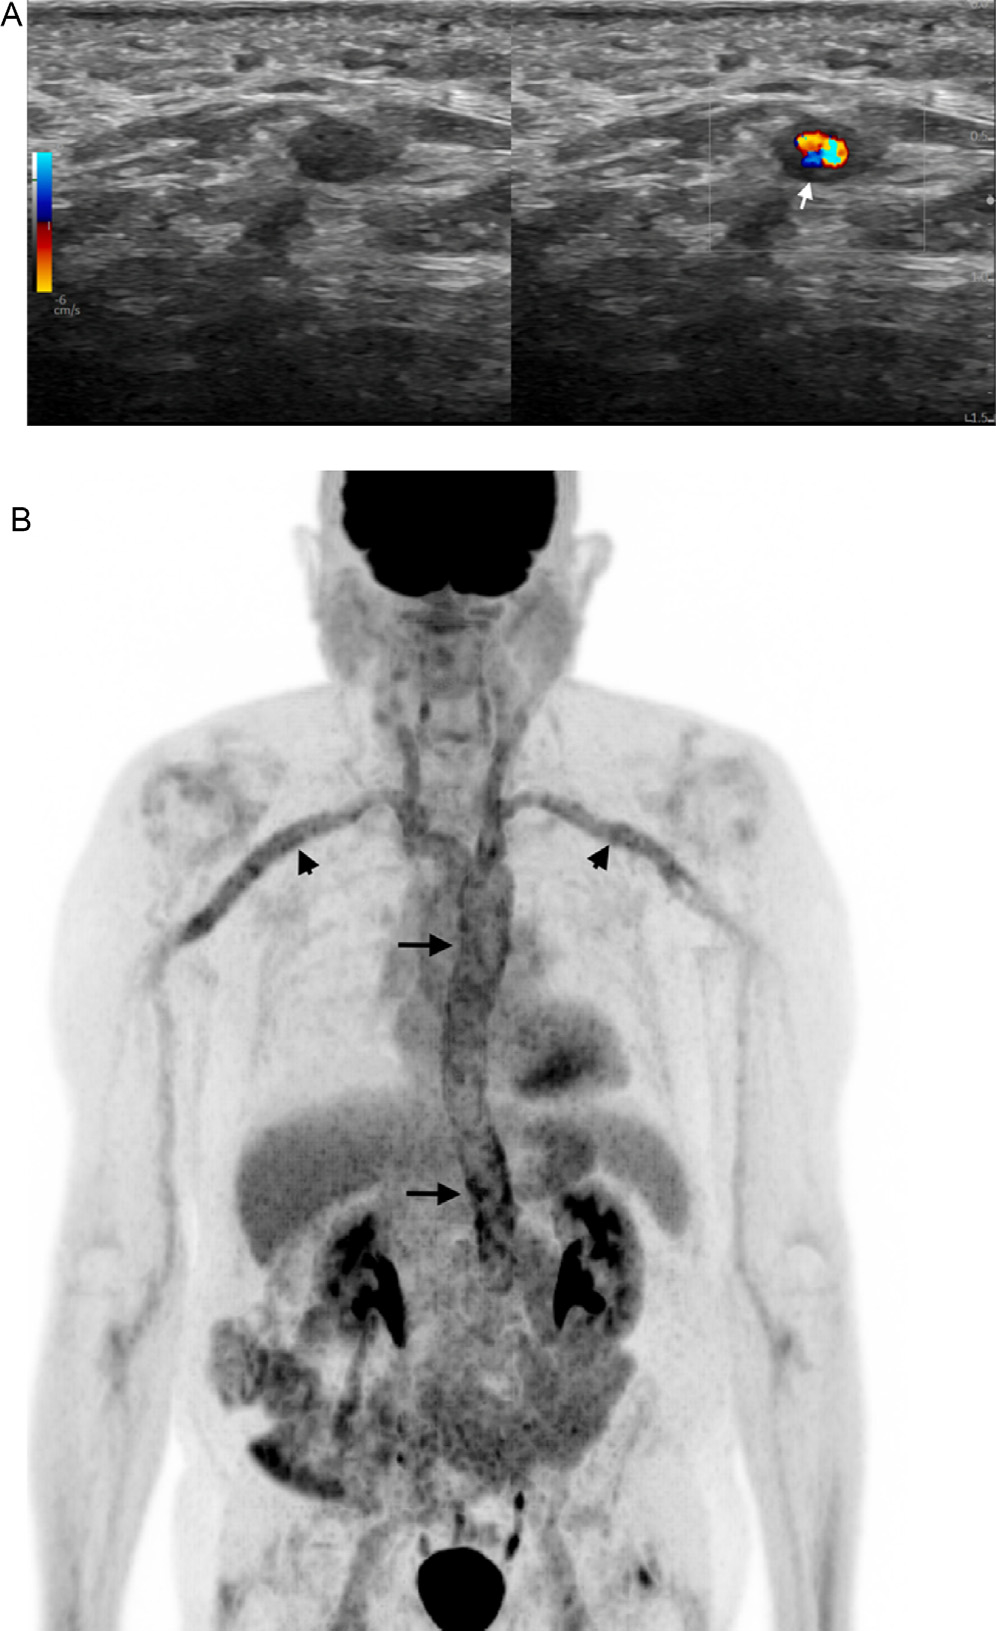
An 85-year-old male presented with fatigue elevated inflammatory markers, underwent US/CDUS of the head and neck (A), which demonstrated circumferential wall thickening (arrow), a classic halo sign of the temporal and supra-aortic arch vessels, including carotid and subclavian arteries, consistent with c-GCA. To assess aorta and the branches, the patient underwent FDG-PET/CT. MIP FDG-PET/CT image (B) showed intense FDG uptake throughout the aorta and supraaortic branches, which confirmed lvGCA. US/CDUS, Ultrasound with Color Doppler; cGCA, cranial GCA; FDG-PET/CT, [18F]-fluoro-2-deoxy-d-glucose positron emission tomography/computed tomography; MIP, maximum intensity projection; lvGCA, large vessel GCA.
